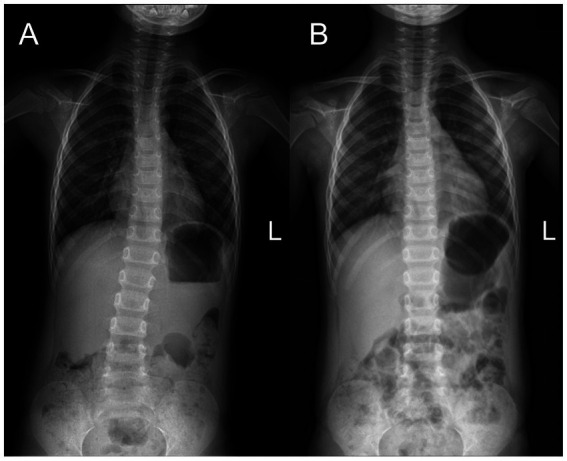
Full-length spinal anteroposterior X-ray radiography in case 2. (A) The Cobb angle was 13° before treatment. (B) The Cobb angle was 0° after 3 months of MSIT treatment.
